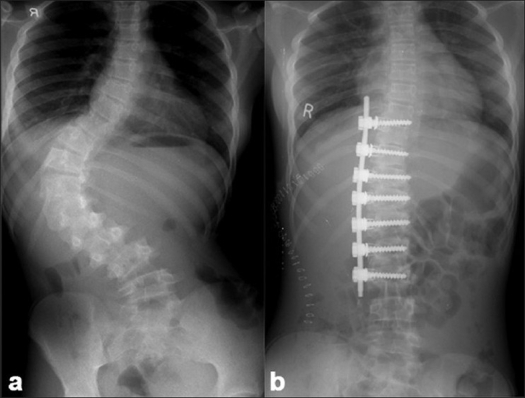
(a) Pre-operative X-ray of dorsal lumbar and sacral spine (anteroposterior view) of a 14 yr old female with Lenke Type 5C right sided thoracolumbar curve. Post-operative radiograph depicting good correction achieved
